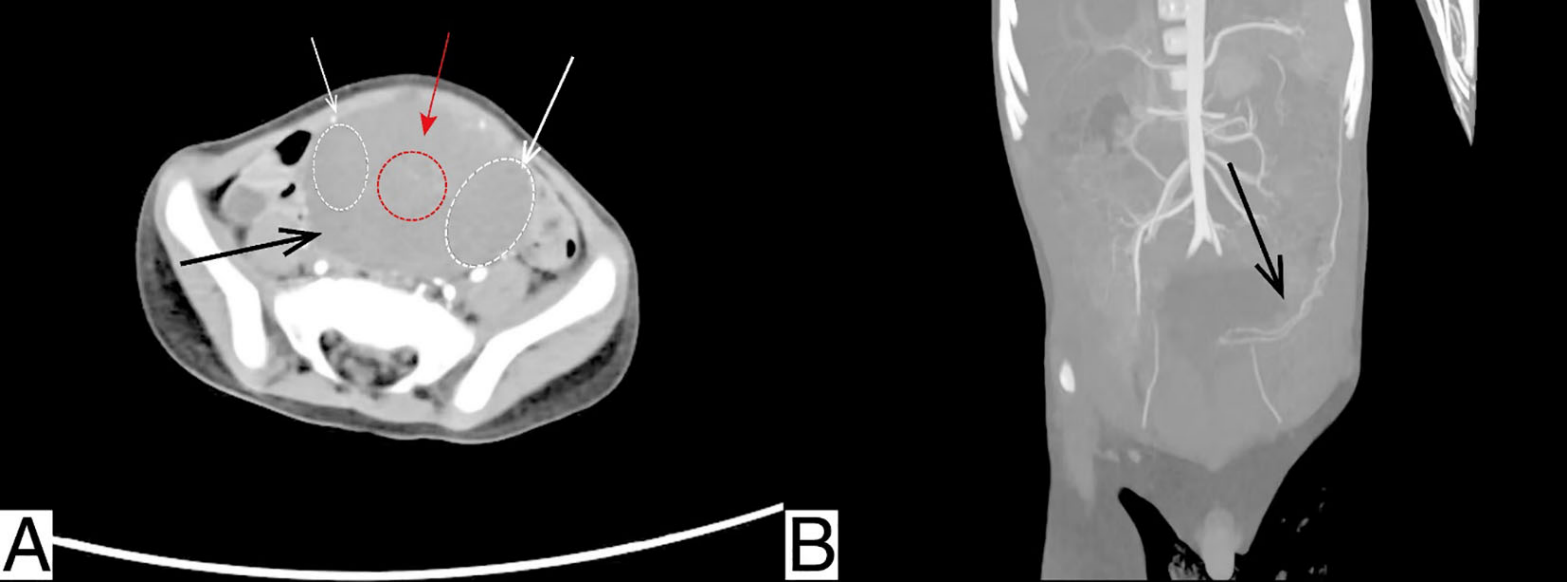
Contrast-enhanced abdominal CT. (A) Contrast-enhanced CT image demonstrates a mixed cystic–solid pelvic mass (black arrow). White arrows and dashed outlines indicate the cystic components, while the solid component, showing heterogeneous progressive enhancement, is indicated by the red arrow and dashed outline. (B) Arterial phase imaging demonstrates the lesion’s feeding artery (black arrow) originating from a branch of the splenic artery and supplying the mass.
